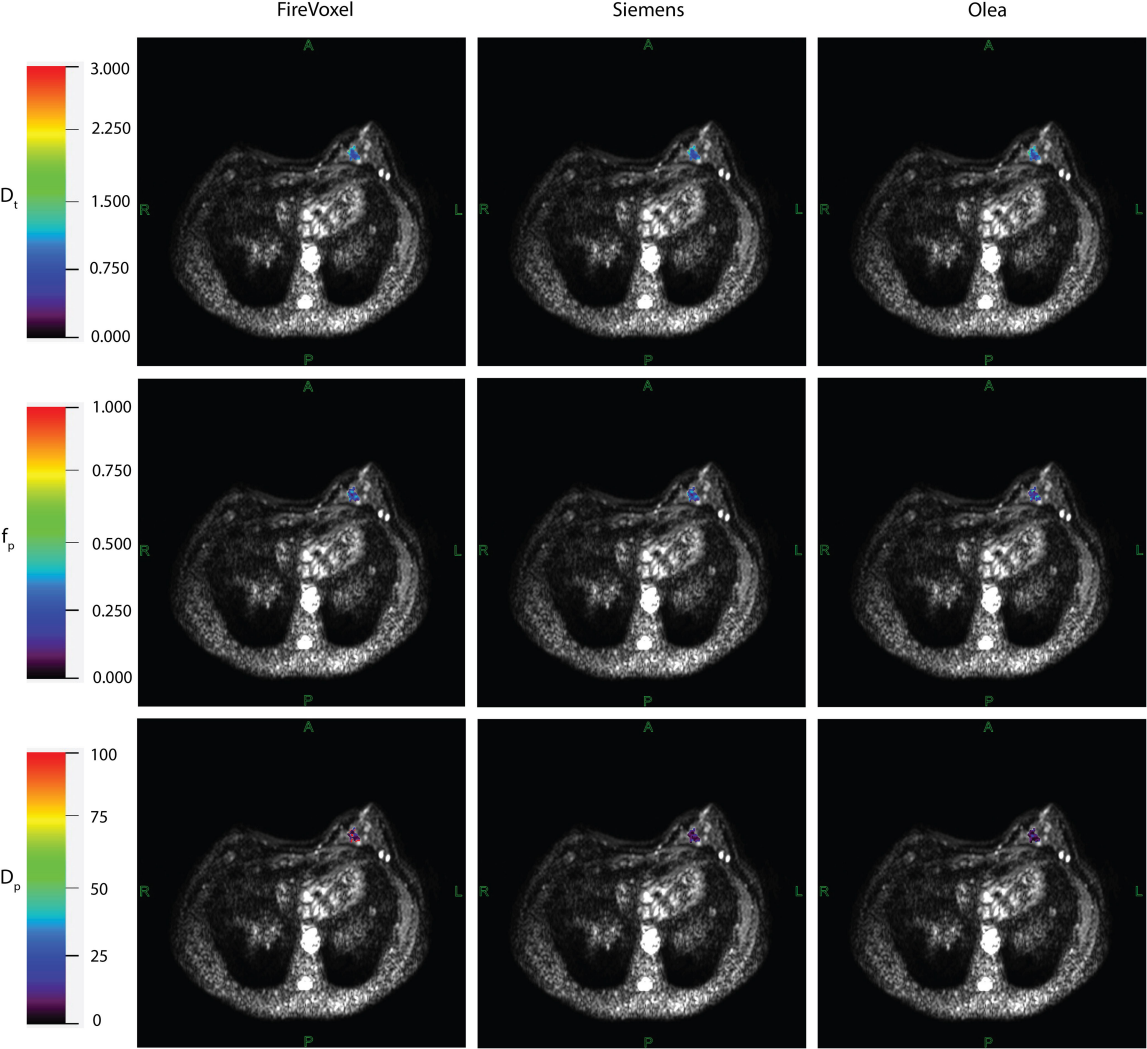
IVIM parametric maps overlaid on raw DWI images in a patient with malignant breast lesion for Site B. IVIM parameters tissue diffusivity (*D_t_
